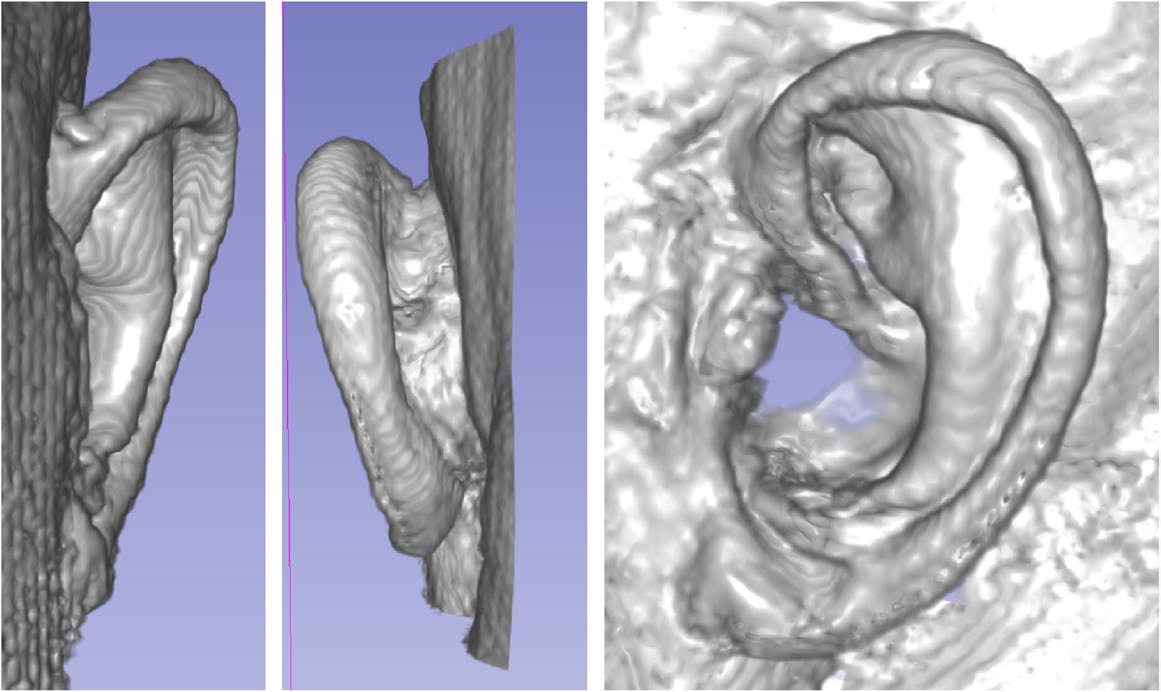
Examples of a 3D reconstruction of a left outer ear from a 3T MRI scan (of author TW) using Slicer 3D software [47]. Left side: posterior-anterior view; center: anterior-posterior view; right side: side-view
